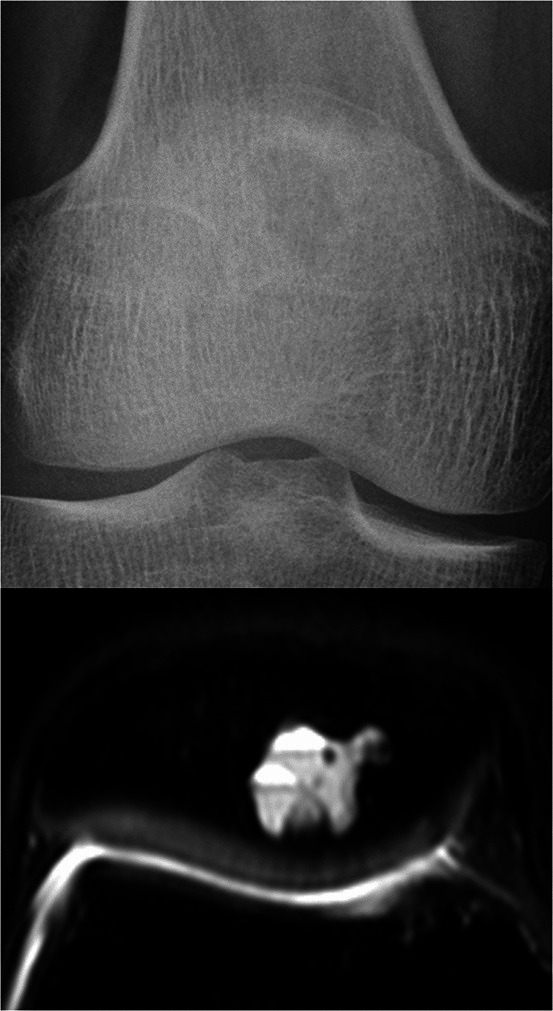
Forty-four-year-old male with patellar chondroblastoma. AP knee radiograph (top) identifies a well-defined lytic lesion within the patella with a well-defined sclerotic margin. Intralesional chondroid matrix mineralisation is difficult to appreciate. Axial PDFS image (bottom) reveals a corresponding lobular lesion with internal fluid–fluid levels. Despite the lack of perilesional oedema, the location suggests ABC-like changes within a chondroblastoma. An epiphyseal lesion containing multiple fluid–fluid levels suggests a pre-existing chondroblastoma or giant cell tumour even without a clear solid component
